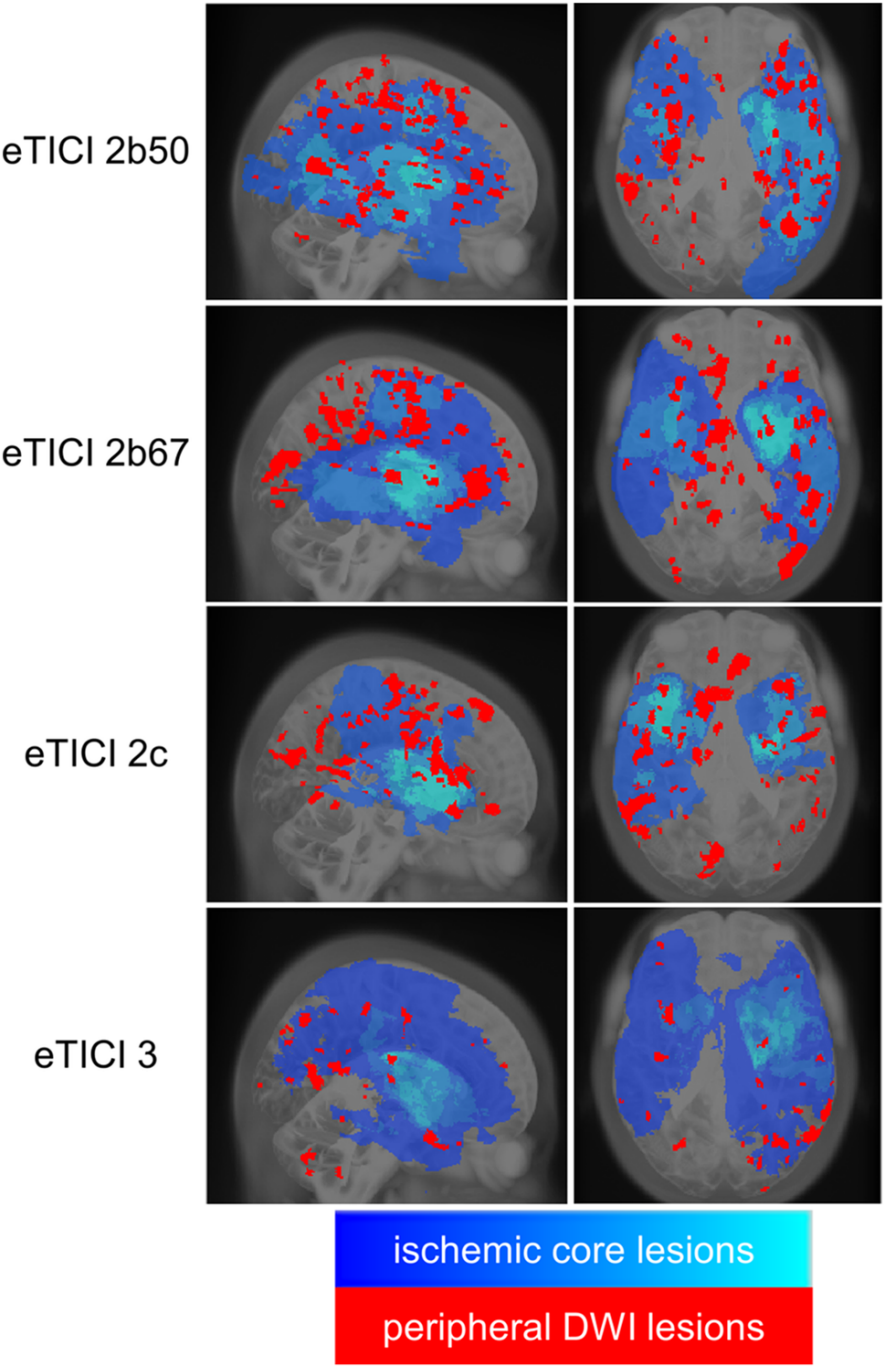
Probability maps of the ischemic core (high probability = light blue; low probability = dark blue) and peripheral DWI lesions (red) in sagittal (left) and axial (right) projection
